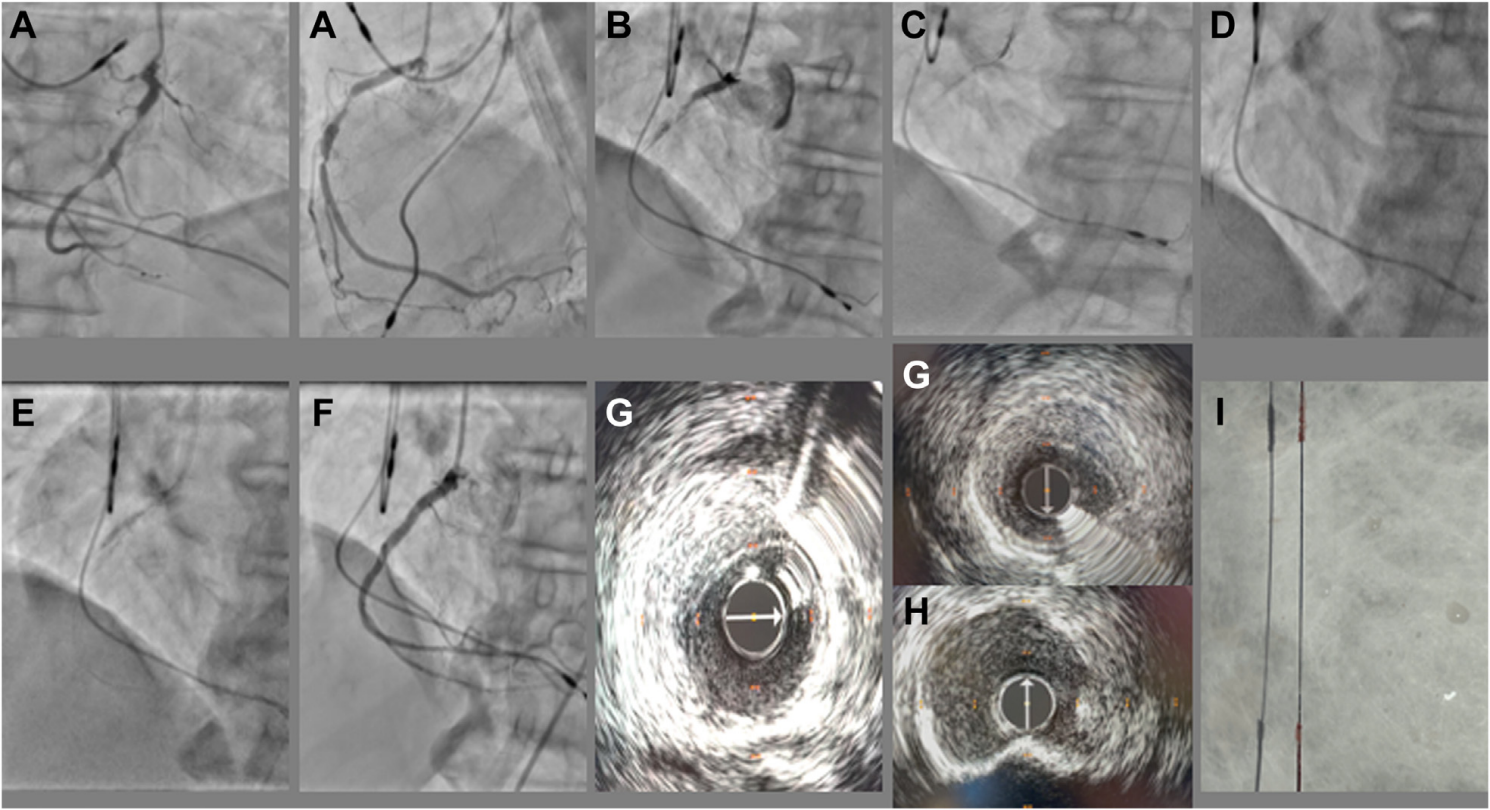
Coronary Angiograms (A) Initial diagnostic angiogram. (B) Ruptured balloon in the proximal right coronary artery (RCA). (C) Unable to advance guidewire. Balloon fragment obstructing the proximal RCA. (D) Subintimal wiring and subintimal tracking and reentry. (E) Subintimal plaque modification, with 2.5-mm noncompliant balloon inflated in the subintimal space, attempting to dislodge entrapped balloon. (F) Final angiographic result. (G) Intravascular ultrasound (IVUS) image demonstrating the crescent-shaped subintimal hematoma. (H) Post angioplasty IVUS demonstrating a calcium nodule in the proximal RCA at the site of balloon entrapment. (I) Ruptured balloon fragments glued to the wire.
